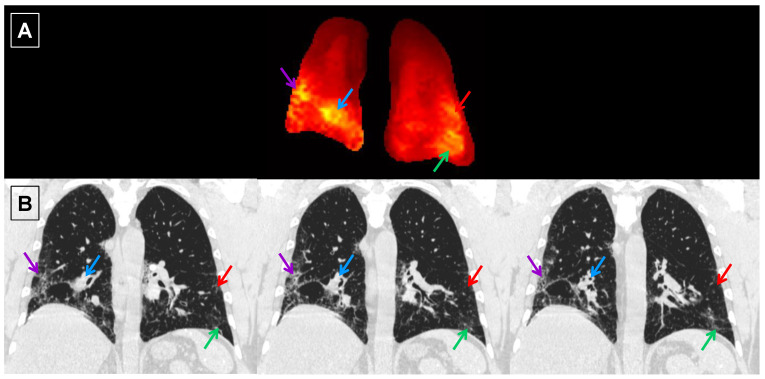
Central coronal slice of the Tissue/RBC maps (A) and spatially matched coronal HRCT images (B) from IPF subject I6. Due to the difference in the slice thickness between the Tissue/RBC maps (15 mm) and HRCT images (0.7 mm), information from multiple nearby HRCT images could appear in a single slice of the Tissue/RBC maps. Each arrow points to a region of high Tissue/RBC, and the same color arrow points to the corresponding region of fibrosis in the HRCT images.
